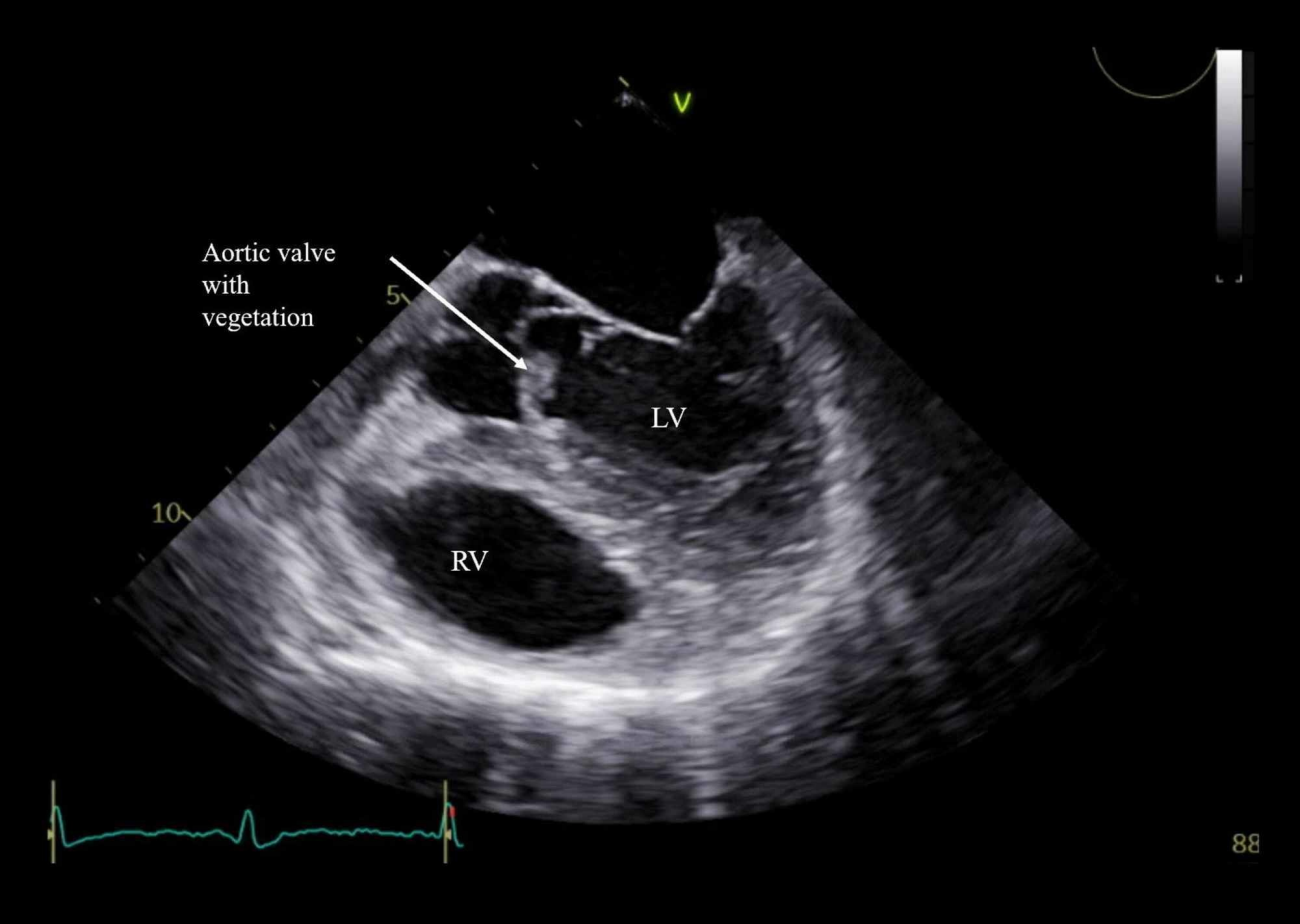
Transesophageal echocardiogram demonstrating vegetation on the aortic valve. LV: left ventricle; RV: right ventricle
